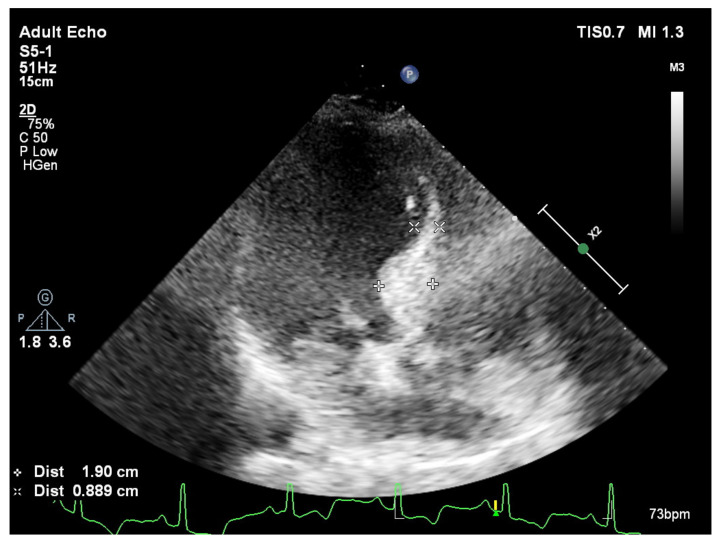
Predominantly placed hypertrophy over septal base from apical 4 chamber view during end-diastole in a patient with aortic stenosis and basal septal hypertrophy.
